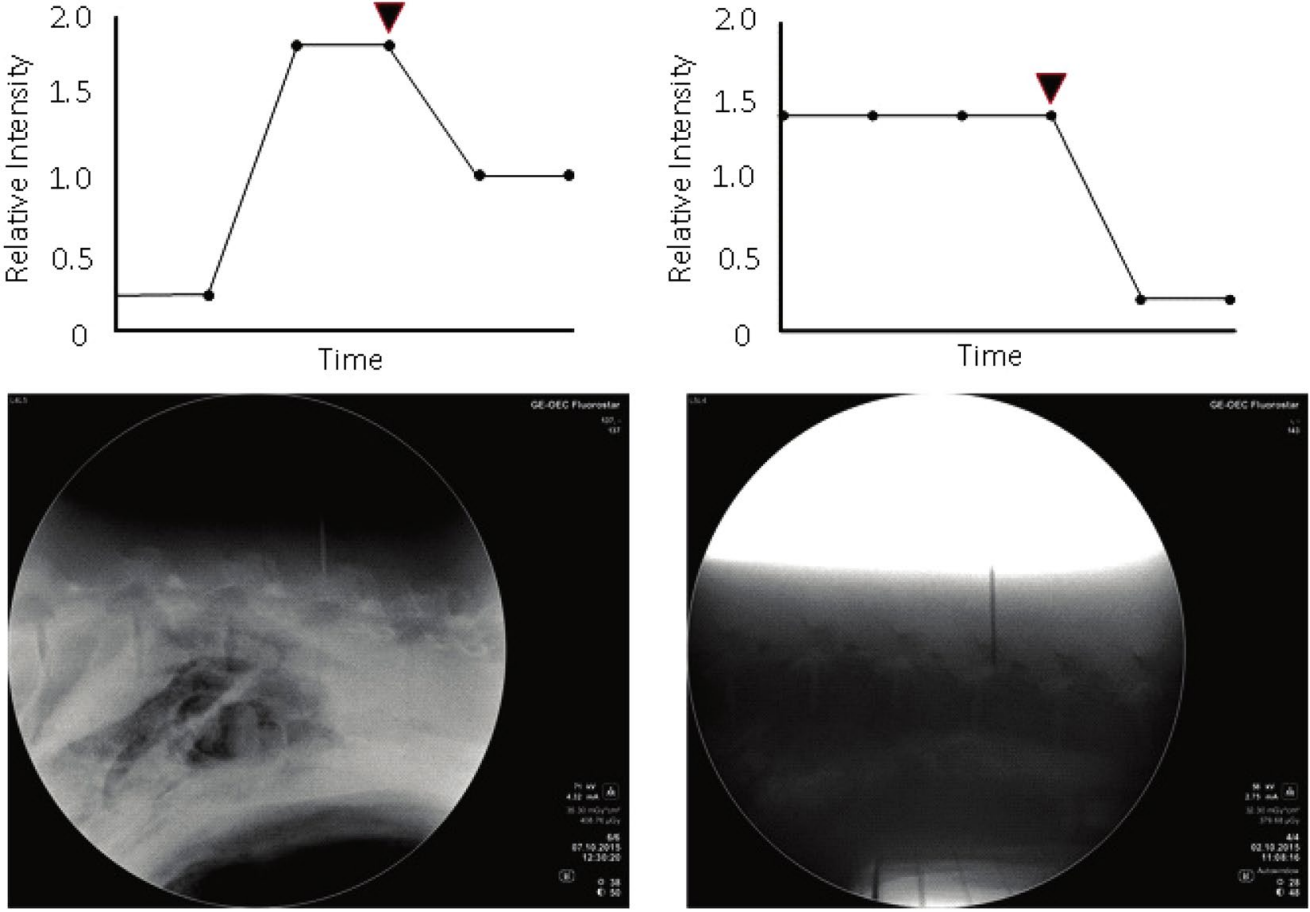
Data change during lumbar puncture using proposed optically guided location device for dural puncture operation. Black triangles indicate timing locating ligamentum flavum. The radiography confirms correctness of needle placement.
